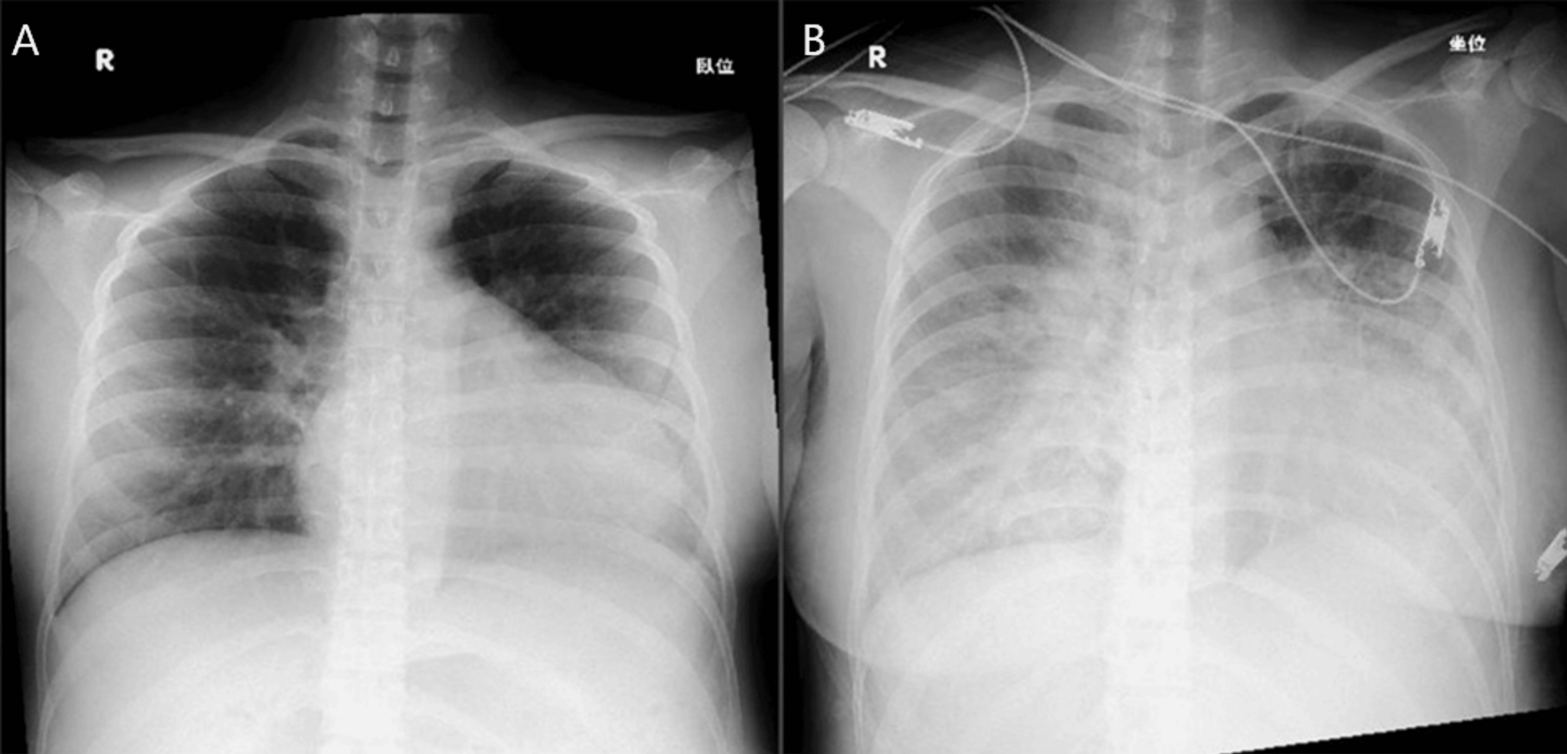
Chest X-ray of the mother A: At the time of admission; B: First day after giving birth
